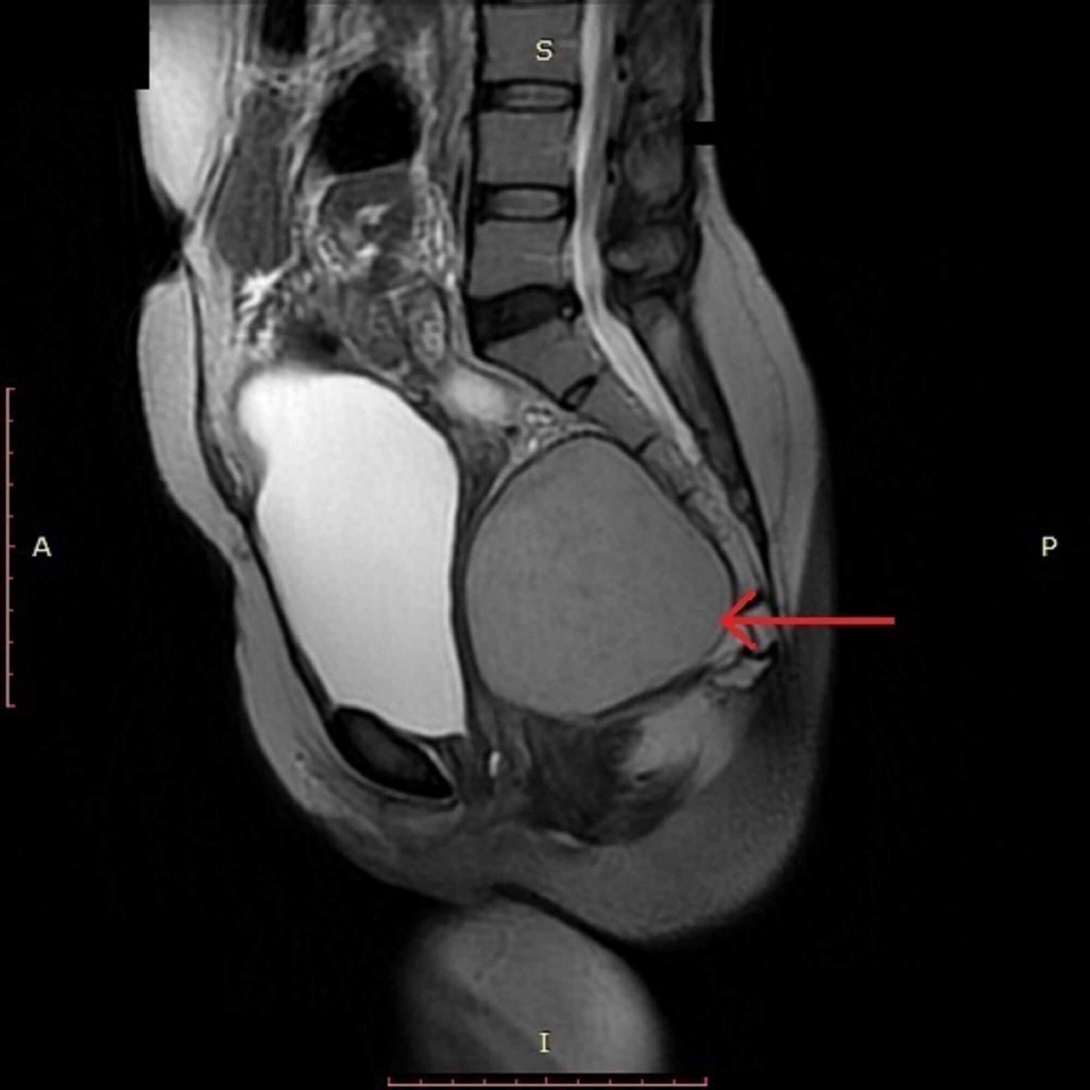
T2W-MRI showing a large, slightly hyperintense lesion in the presacral space T2W, T2-weighted; MRI, magnetic resonance imaging
